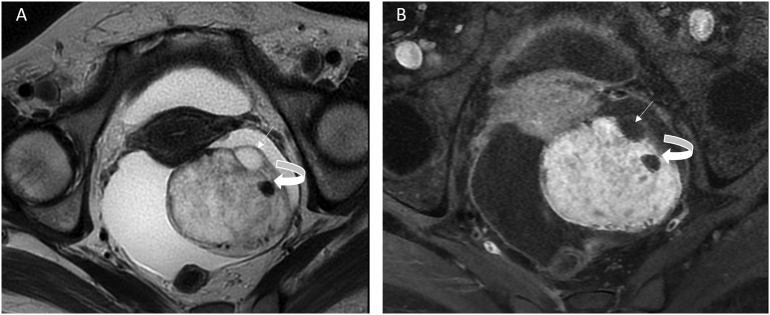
(A&B) T2-weighted and post contrast fat-sat T1-weighted showed a very low signal intensity (curved arrow), due to the viscous colloid material that could be suggestive for the presence of struma ovarii tumor. Thin arrow = follicule.
